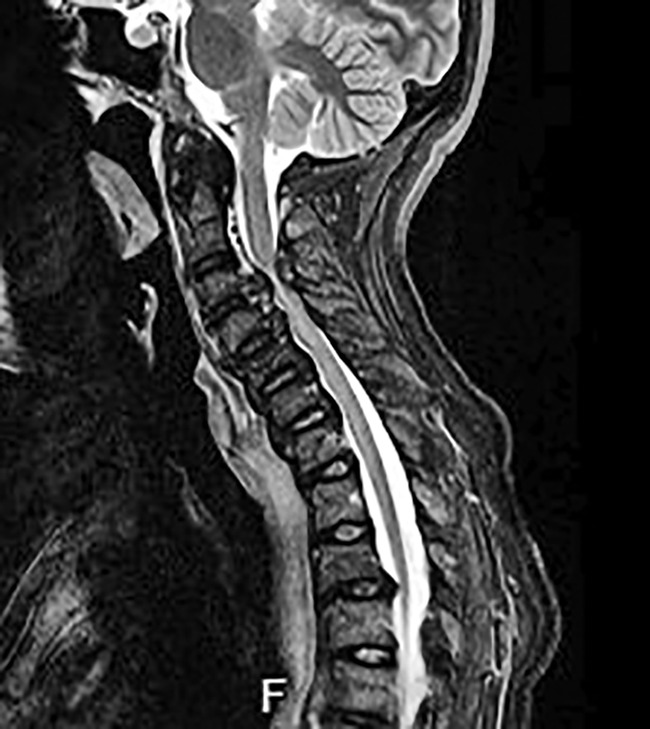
MRI of the cervical spine (December 2018). Sagittal sections, T2‐weighted sequence: Increased ossifications of the posterior longitudinal ligament and the yellow ligaments responsible for narrowing of the vertebral canal, which causes spinal cord compression, as shown by the presence of a C_3_–C_4_ intramedullary hypersignal.
